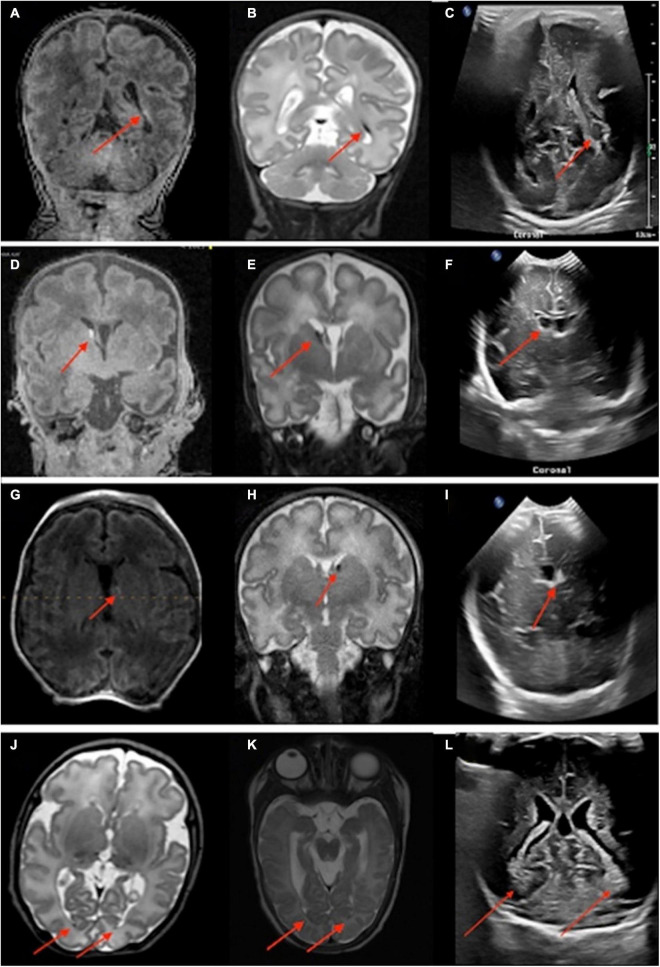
Neonates scanned on point-of-care 1-Tesla MRI for intraventricular hemorrhage (IVH) follow-up. An 80-day-old infant with T1 hyperintense (A) and T2 hypointense (B) grade II left germinal matrix hemorrhage on point-of-care 1-Tesla MRI performed 70 days after transcranial ultrasound (C). A 52-day-old infant with T1 hyperintense (D) and T2 hypointense (E) right-sided grade I germinal matrix hemorrhage on point-of-care 1-Tesla MRI performed 34 days after transcranial ultrasound (F). A 28-day-old infant with T1 hyperintense (G) and T2 hypointense (H) left-sided grade I germinal matrix hemorrhage on point-of-care 1-Tesla MRI performed 15 days after transcranial ultrasound (I). An 85-day-old infant with layering T2 hypointense IVH (J) on point-of-care 1-Tesla MRI, which was also visualized on follow-up 3-Tesla MRI 38 days later (K) and was sequela of grade 2 IVH seen on transcranial ultrasound performed 74 days earlier (L).
